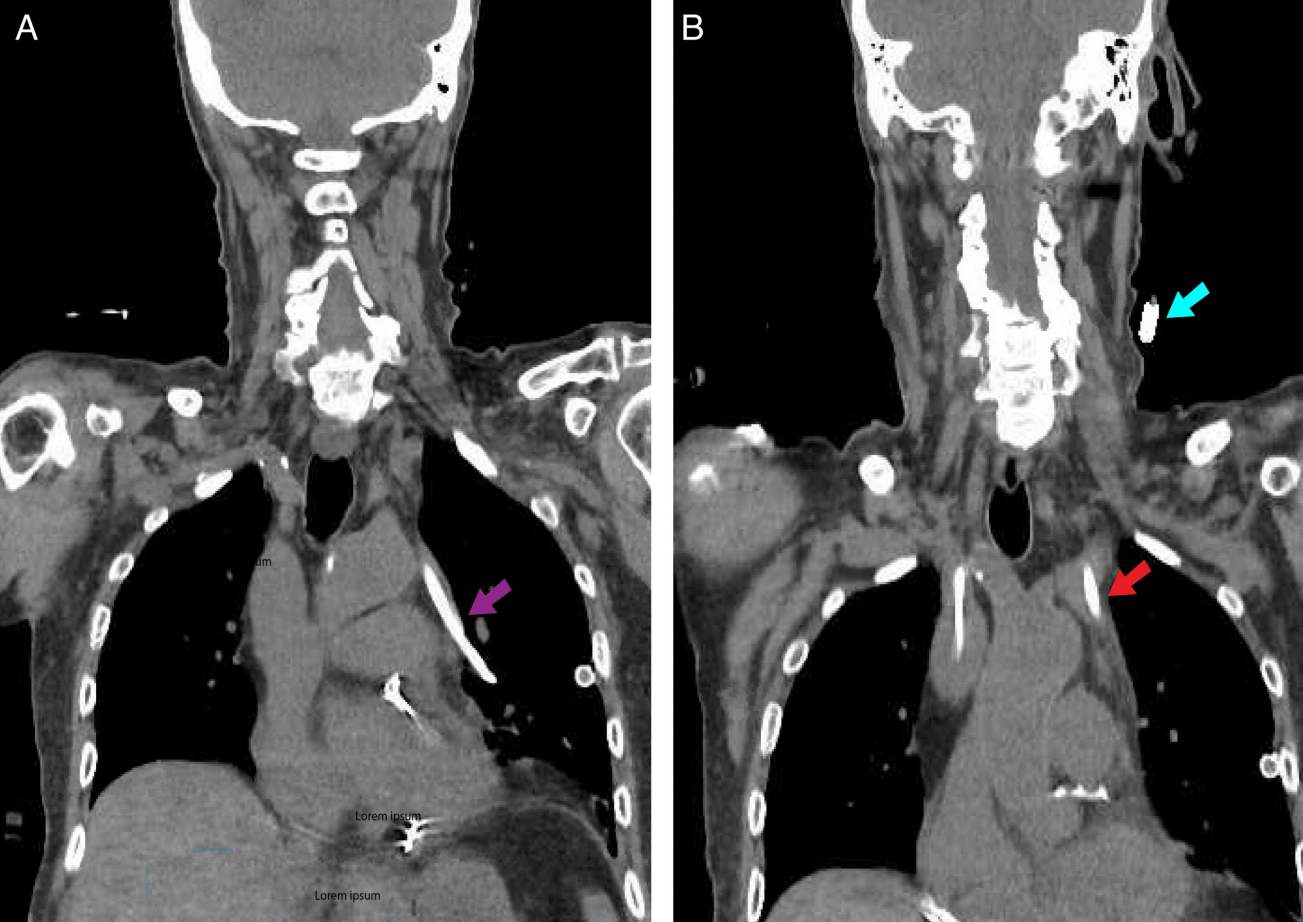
CT neck and chest without contrast showing central line visible in anomalous left upper lobe pulmonary vein CVC placed in partially anomalous pulmonary venous return (PAPVR) (A) CT imaging confirming the catheter was inadvertently placed in an anomalous left pulmonary vein (purple arrow). (B) The blue arrow showing proximal catheter entry point into a left internal jugular. The red arrow tracking the insertion of the pulmonary vein to the left innominate vein. CVC: Central venous catheter
